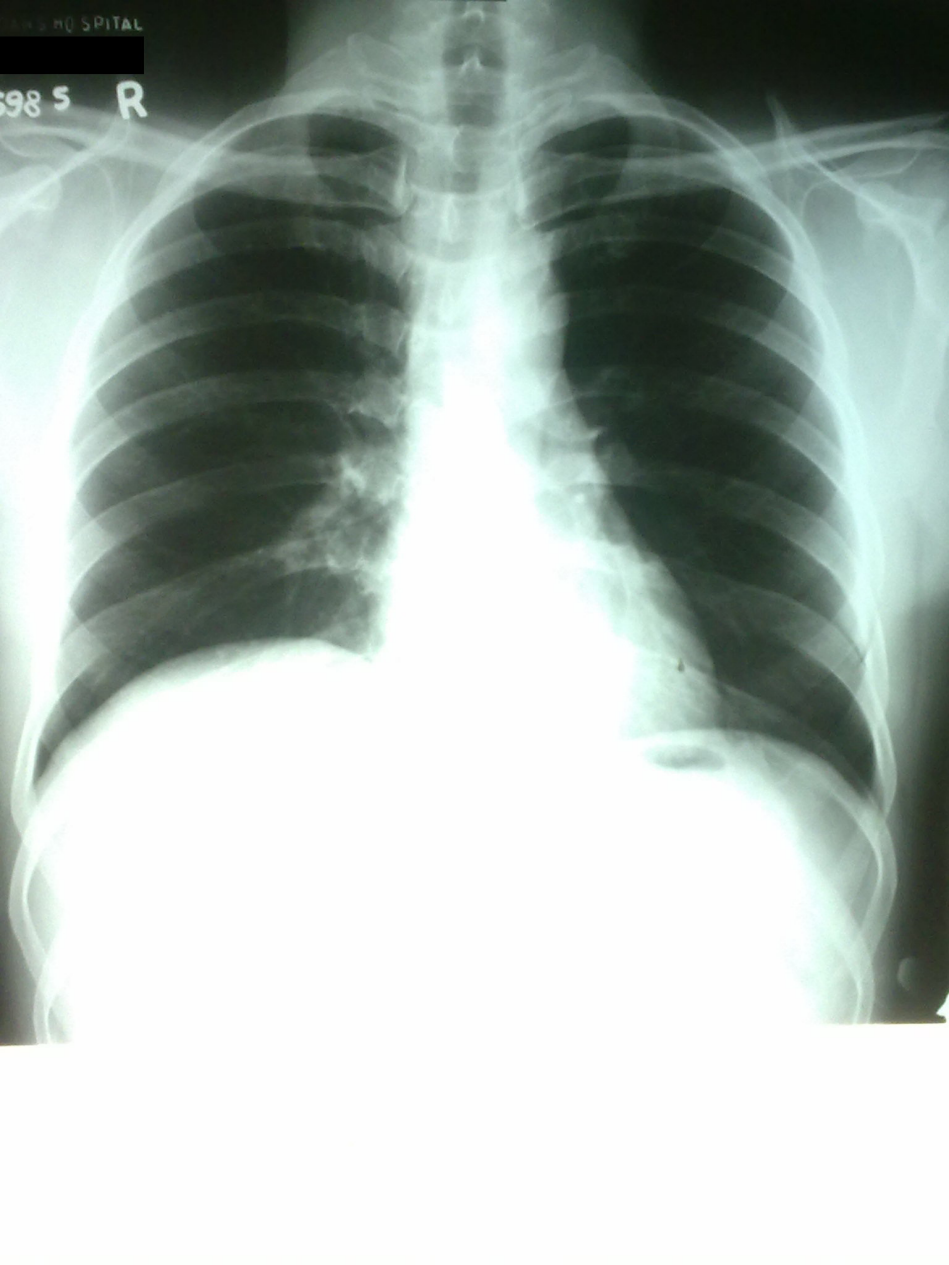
Chest X-Ray two weeks after second discharge.
